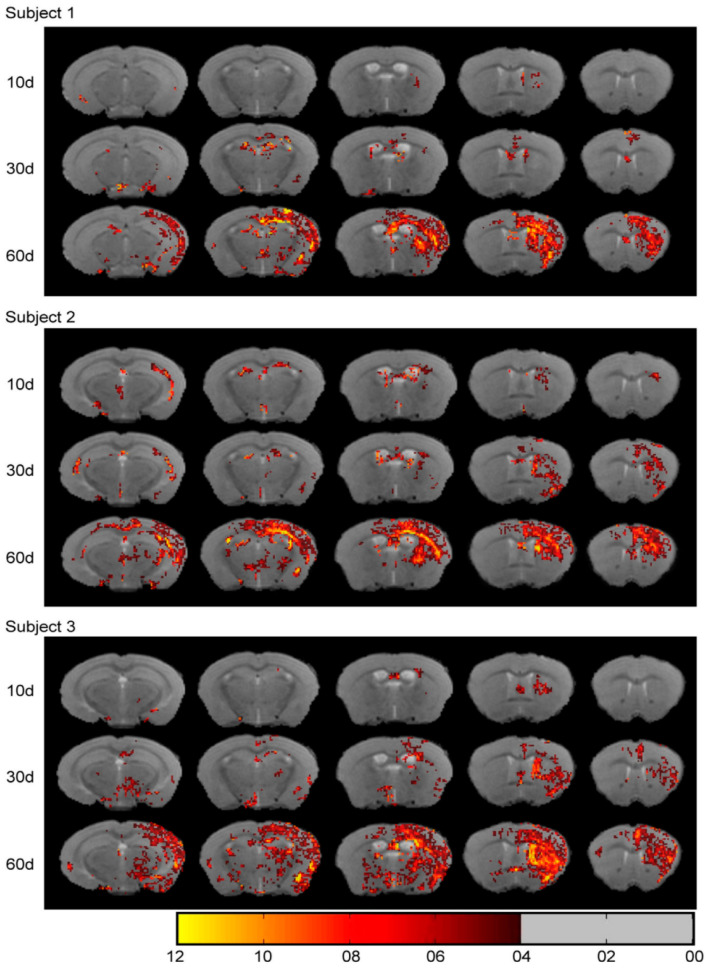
After different times (10 days, 30 days, or 60 days) of the rAAV2-retro–CAG–Ferritin injection, the CPU connection area is displayed by MRI in vivo (expressed by the change in T2 relaxation times). A color change (red–yellow) is used to indicate a change in T2 relaxation time (4–12 ms). Reproduced with permission from Ref. [33], 2021, Wiley.
